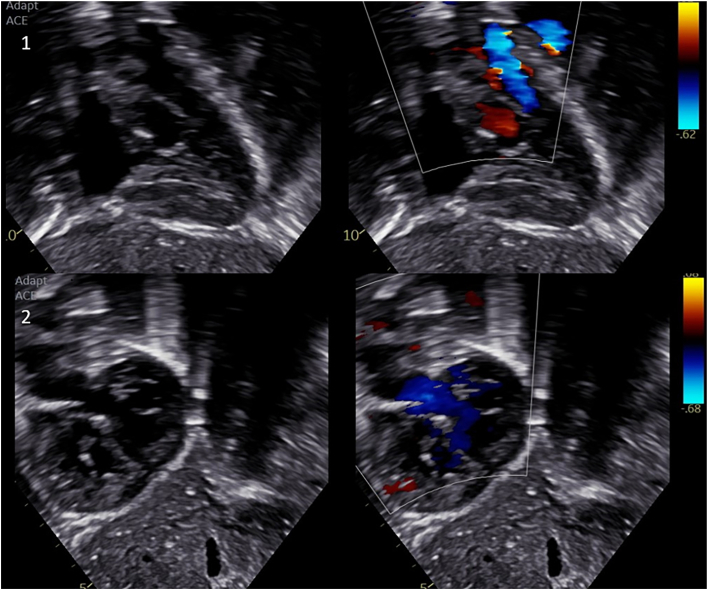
Postoperative echocardiography shows the (1) right ventricular outflow tract and (2) left ventricular outflow tract.
